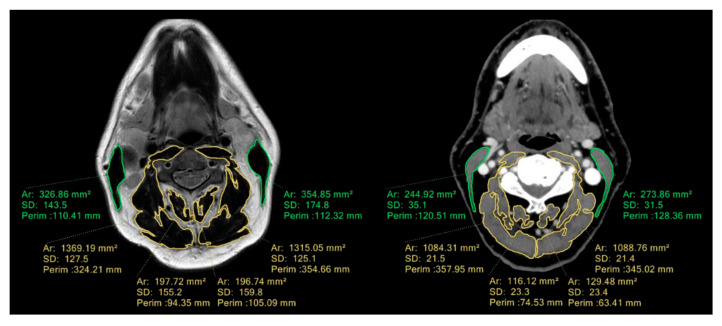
Segmentation of sternocleidomastoid (green) and paravertebral muscles (yellow) at C3 level using MRI (left) and CT (right) imaging in two different patients.
